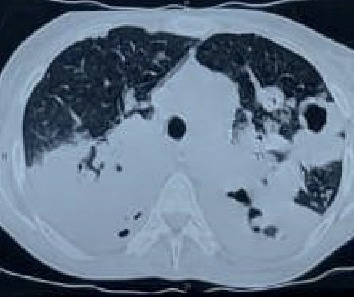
Axial section of CT thorax showing bilateral consolidation with necrosis and cavitation.
